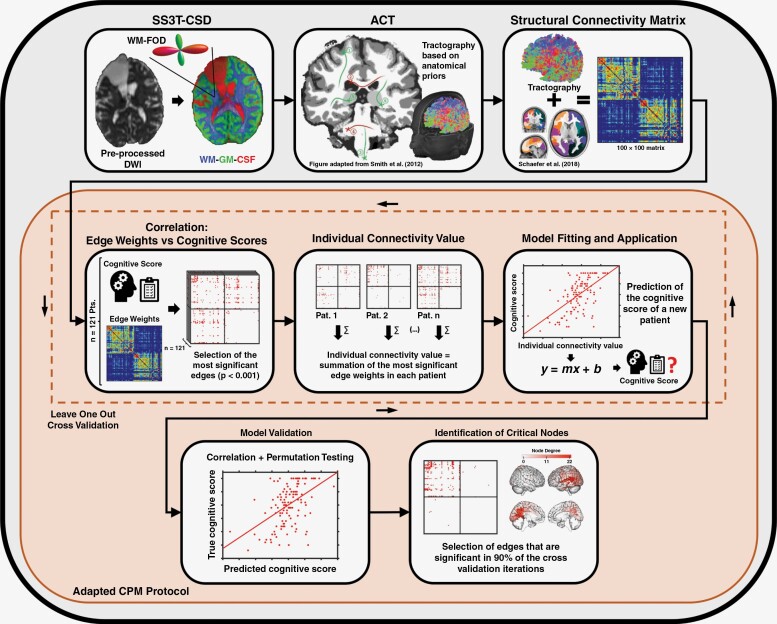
Overview of methods for determining the whole-brain structural connectome and generating and validating a connectome-based predictive model (CPM) for cognitive functioning in glioma patients following multimodality treatment. SS3T-CSD, single-shell 3-tissue constrained spherical deconvolution; WM, white matter; FOD, fiber orientation distribution function; DWI, diffusion-weighted magnetic resonance imaging; GM, gray matter; CSF, cerebrospinal fluid; ACT, Anatomically Constrained Tractography; Pts, patients; Pat, patient.
